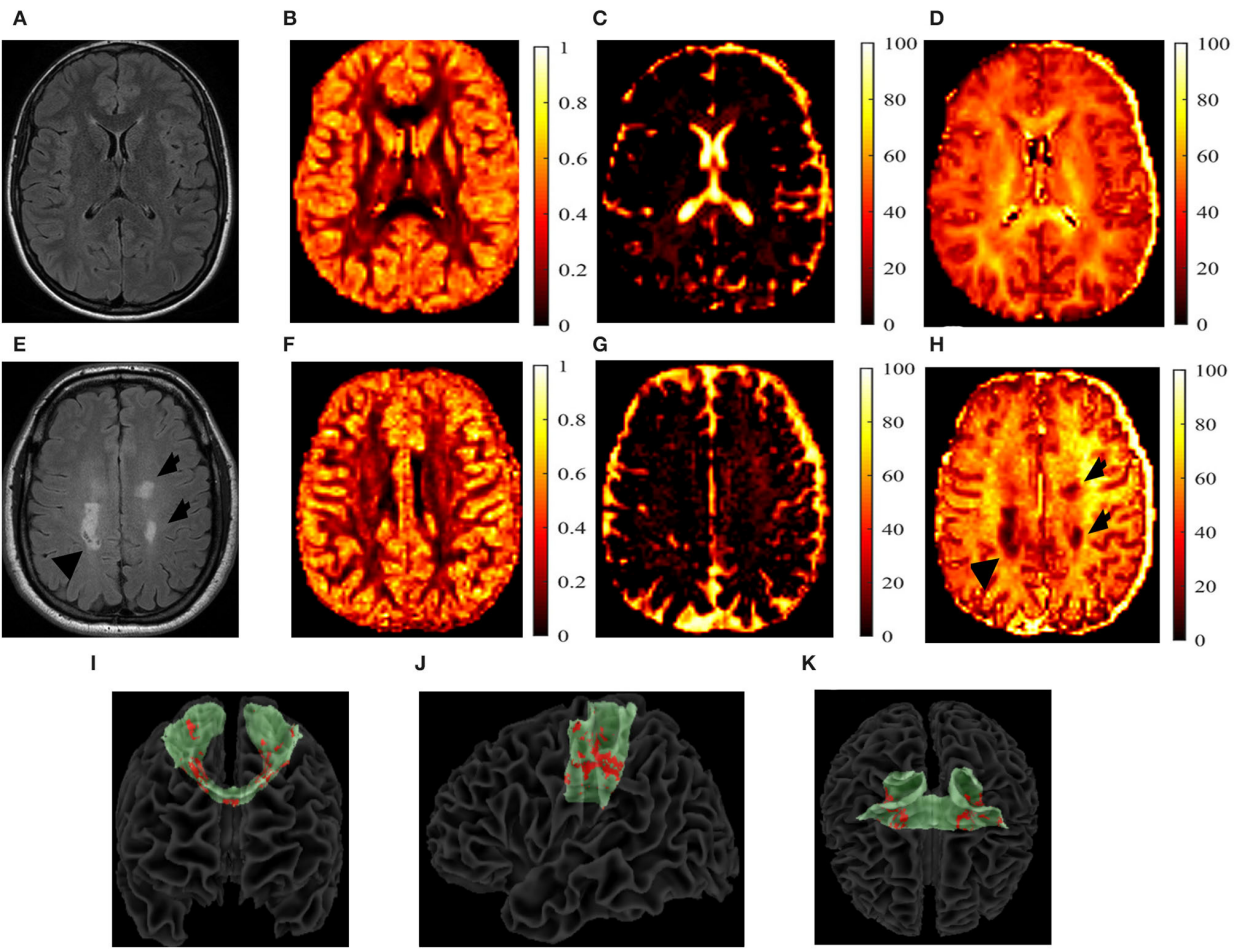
T2-fluid-attenuated inversion recovery (FLAIR) images and the neurite orientation dispersion and density imaging (NODDI) parametric maps. T2-fluid-attenuated inversion recovery (T2-FLAIR) images and the neurite orientation dispersion and density index (NODDI) parametric maps of a 27-year-old, white, female healthy control (HC) (A–D) and a 40-year-old white person with relapsing-remitting multiple sclerosis (E–H). The T2-FLAIR (A,E), orientation dispersion index (ODI) (B,F), isotropic volume fraction (IVF) (C,G), and the neurite density index (NDI) (D,H) are shown. T2 lesions are indicated by black arrows. Reconstructed transcallosal fibers of the primary motor cortex are shown in green with both the T1 and T2 lesions shown in red with a coronal (I), sagittal (J), and axial (K) views. I-K show the coronal (I), sagittal (J), and axial (K) views of the reconstructed TC fibers of primary cortex in green, with lesions marked in red of a 56-year-old white male with secondary-progressive multiple sclerosis.
